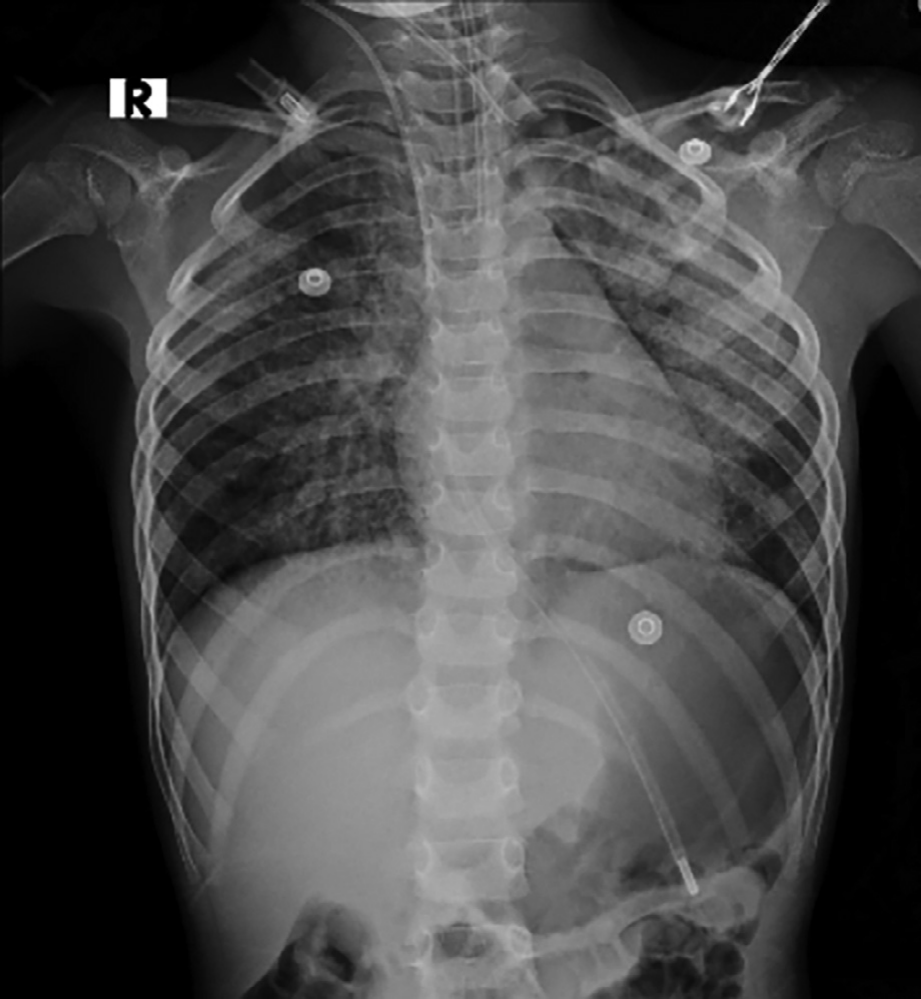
Chest radiograph (X-ray) post right whole lung lavage (WLL) showing reduction of the opacities in the right lung in comparison to the first X-ray in Fig. 1.
